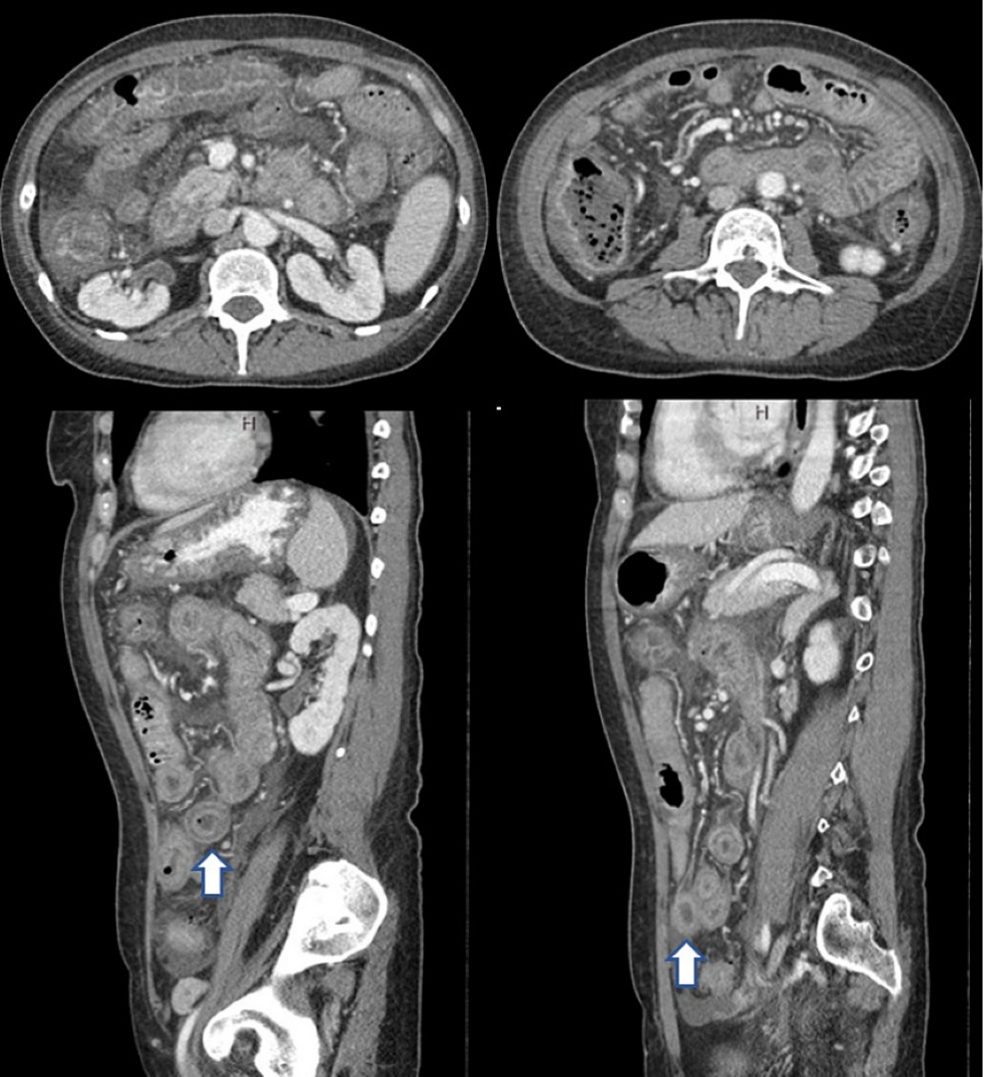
Contrast-enhanced abdominal CT scan - case 2 Diffuse thickening of the small intestine and colon wall with target sign seen (arrows) CT: computed tomography
